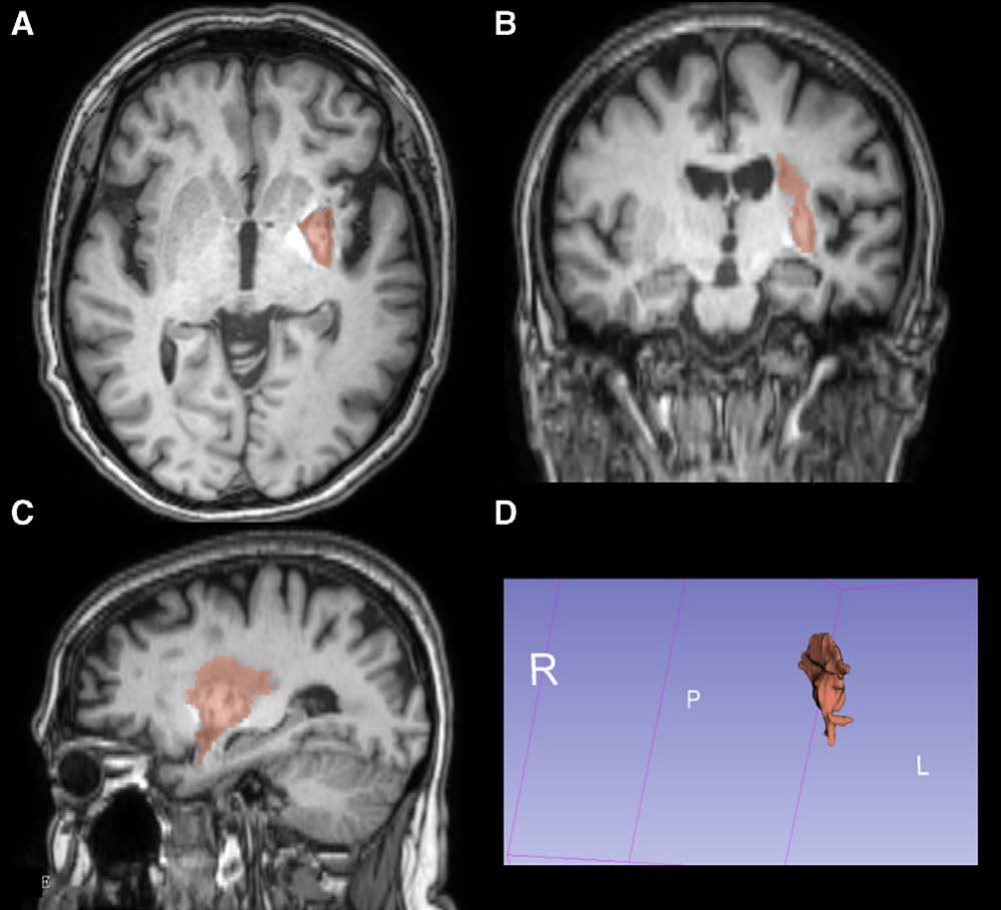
In FLAIR sequence. (A). Axial orientation; (B). Coronal orientation; (C). Sagittal orientation; (D). Segmentation of lesion.
